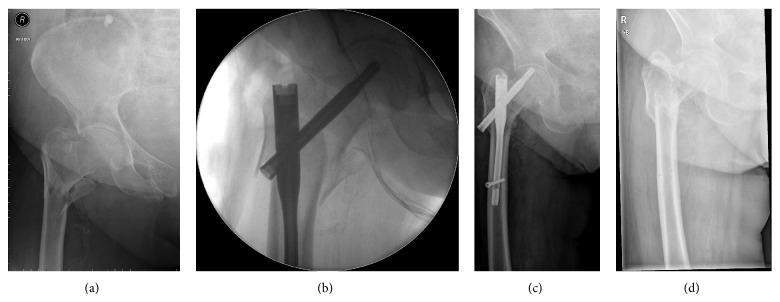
(a) 90-year-old female with proximal femoral fracture (AO/OTA type 31-A2). (b) Intraoperative radiograph anteroposterior view. (c) 5 months after surgery showing the cutout. (d) Immediately after removal of the nail.
